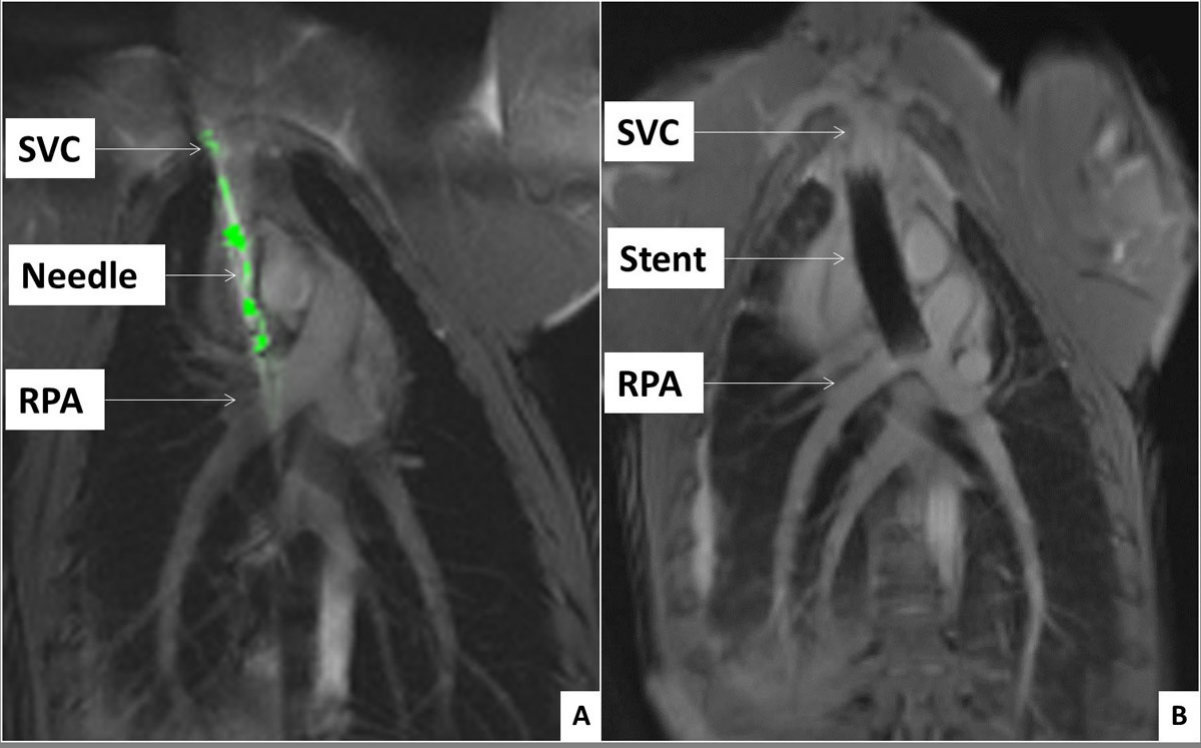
A depicts real-time MRI needle access of adjacent vessels [superior vena cava (SVC) and right pulmonary artery (RPA)]. Figure 1B shows final bidirectional Glenn shunt with anastomosis of SVC and RPA using endograft.
